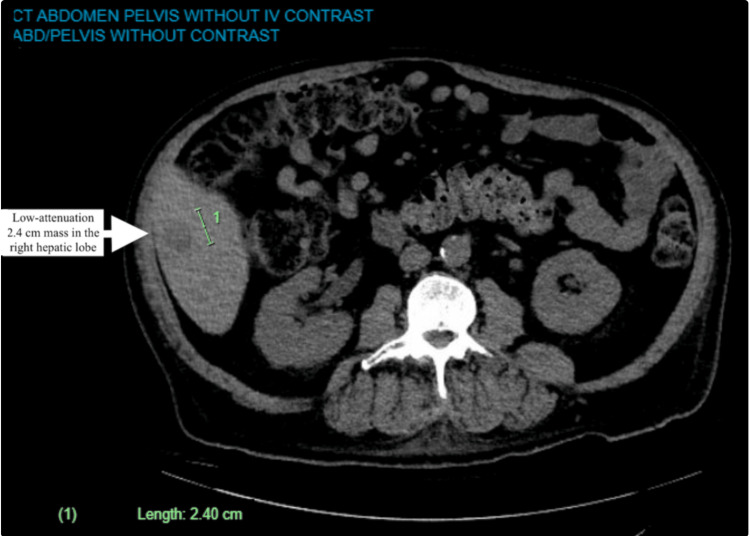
CT scan of the abdomen, with the white arrow showing the initial mass size
